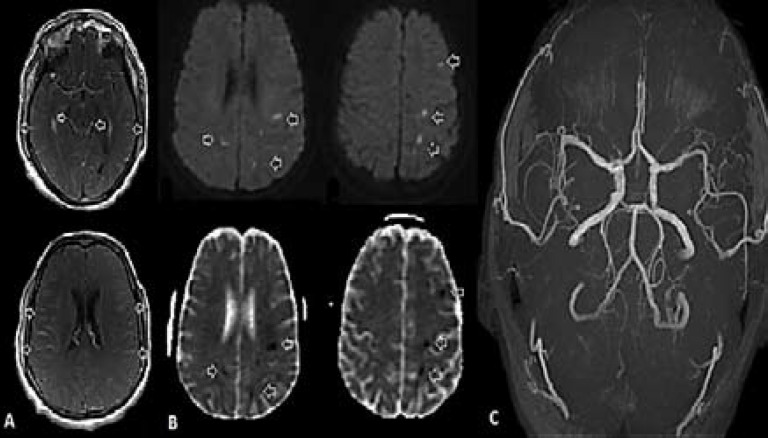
Axial post-contrast magnetic resonance imaging (MRI) images of brain demonstrate leptomeningeal enhancement in bilateral cerebral hemispheres and in basal cisterns (A); axial diffusion-weighted imaging (DWI) images and corresponding axial apparent diffusion coefficient maps demonstrate multiple foci of restricted diffusion in subcortical and deep white matter in bilateral cerebral hemispheres more on left side (B), and axial MRA image demonstrates no evidence of intracranial arterial stenosis/thrombosis (C).
